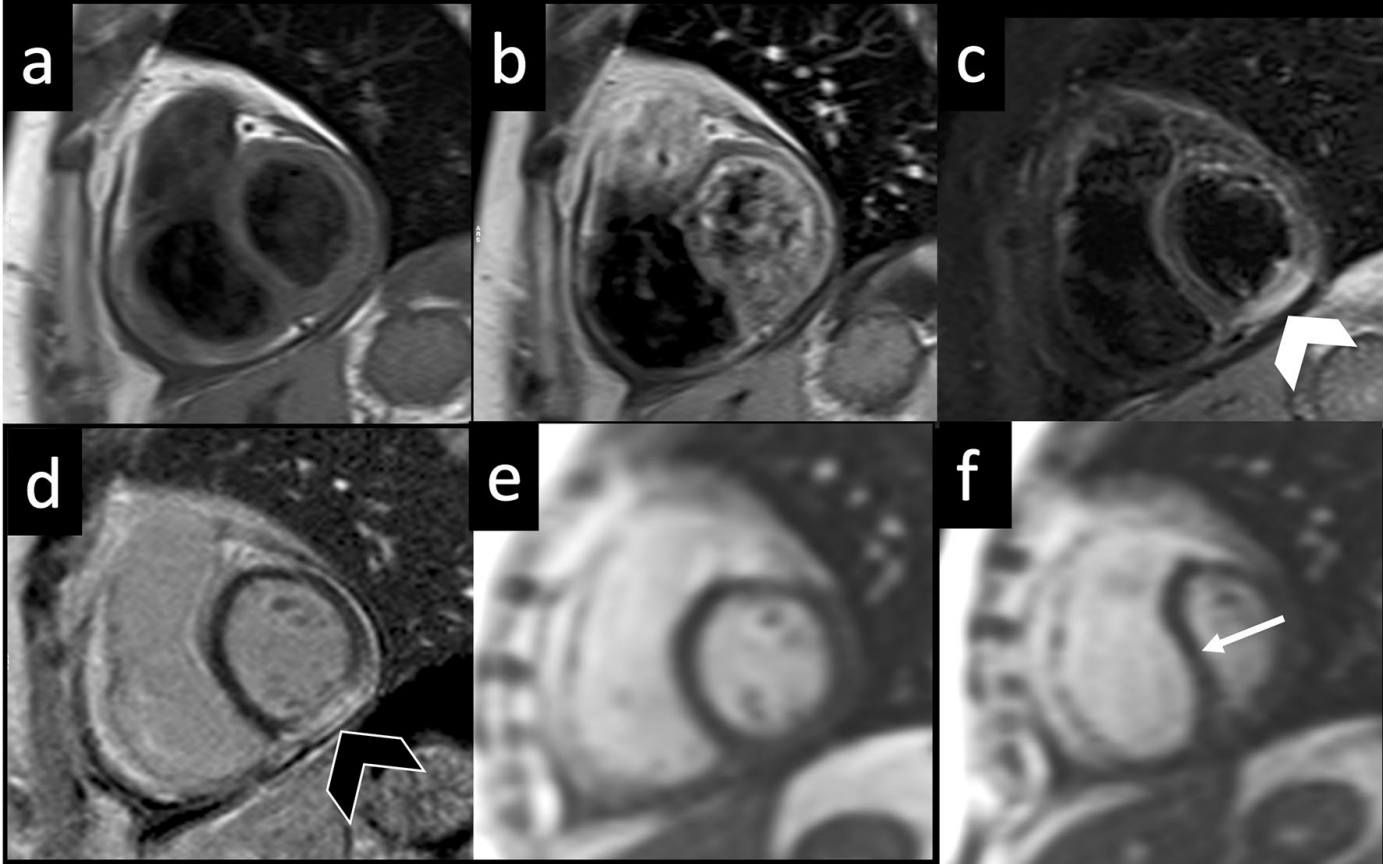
Constrictive pericarditis—TSE T1-weighted images acquired on short axis view before (a) and 3 min after gadolinium administration (b) demonstrate a diffuse thickening of pericardial layer with minimal effusion and slight enhancement of the visceral pericardial layer. On STIR T2-weighted image (c), a focal area on myocardial edema (white arrowhead) is found in the LV inferior wall corresponding to the area of pathological enhancement (black arrowhead) on LGE image (d) by demonstrating a condition of active myocarditis. On free-breathing real-time cine-SSFP acquired in end-expiration (e) and end-inspiration (f), a bouncing and leftward shifting of IVS is seen during inspiration (white arrow) due to the inversion of interventricular pressure ratio combined with inextensibility of the pericardial sac. *TSE* turbo spin echo; *STIR* short tau inversion recovery; *LGE* late gadolinium enhancement; *LV* left ventricle; *SSFP* steady-state free precession; *IVS* interventricular septum
